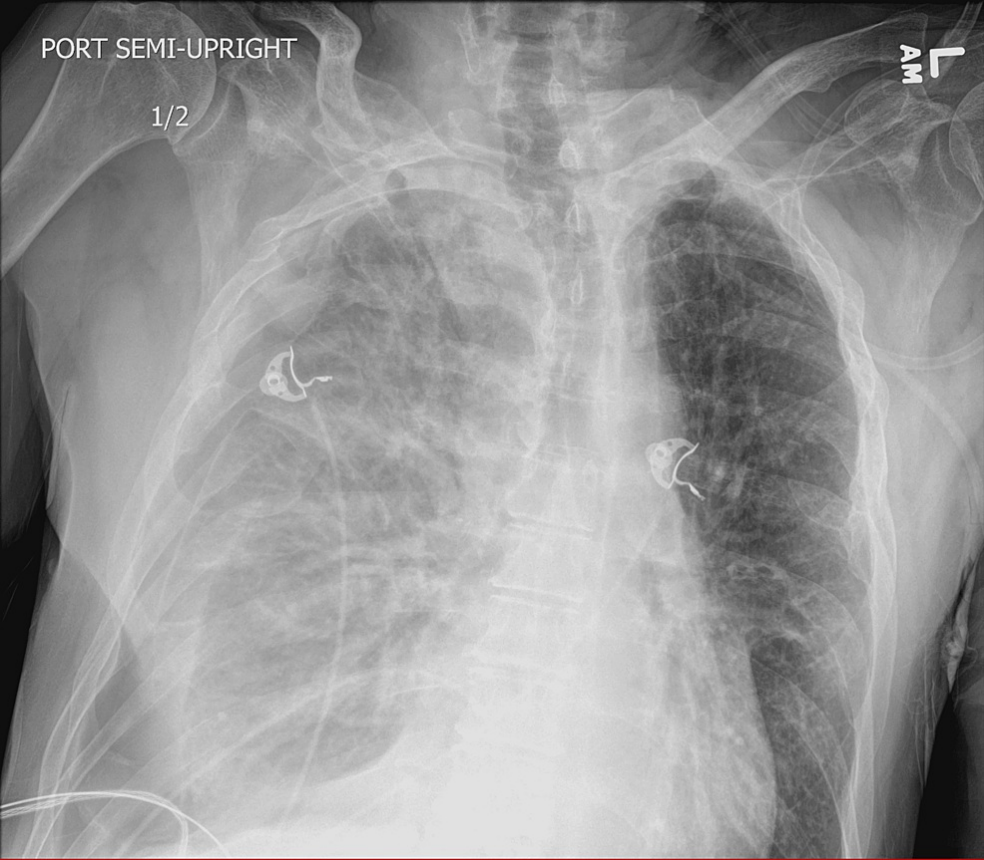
Initial chest x-ray showing right pneumothorax.
